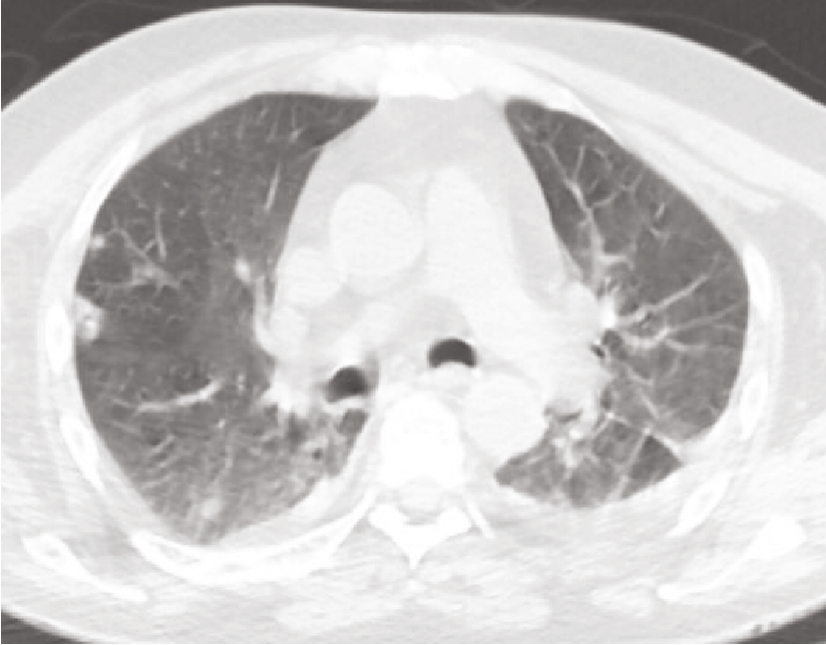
Chest CT scan with multiple irregularly nodular formations.
